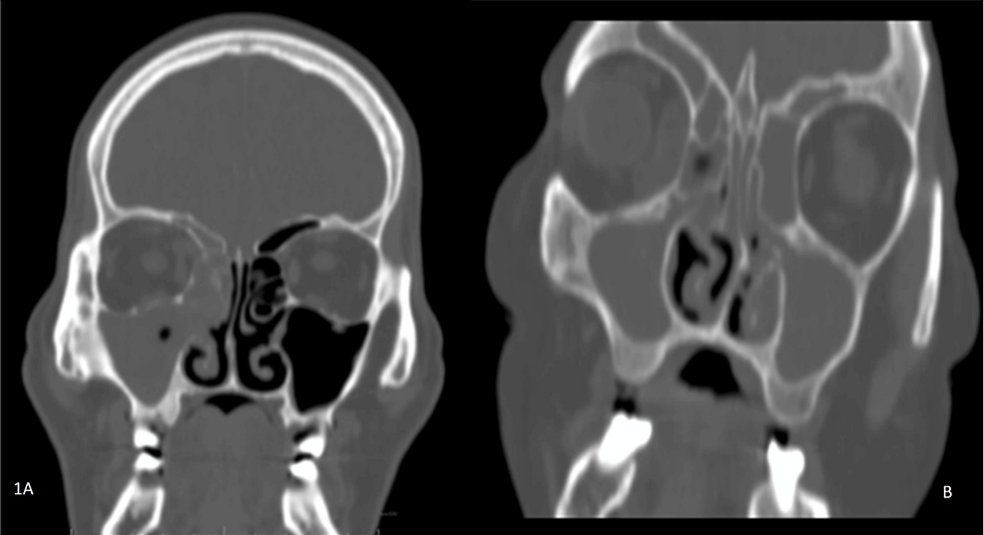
Coronal CT scan images of paranasal sinuses in the bone window depict two male patients aged 48 years and 50 years. (A) Unilateral pansinusitis on the right side shows opacification of the right maxillary sinus, ethmoid sinus, and frontal sinus with the widening of the osteomeatal complex, erosion of the uncinate process, middle turbinate, and bony lamellae of the ethmoid sinus. (B) Bilateral pansinusitis demonstrates opacification of the bilateral maxillary sinus, ethmoid sinus, and frontal sinus.
